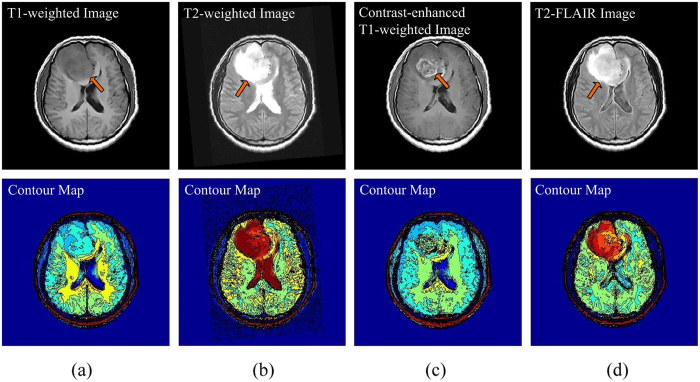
Representative brain tumor MR images under different imaging modes (upper) and the corresponding contour maps (lower). (a) T1-weighted image. (b) T2-weighted image. (c) Contrast-enhanced T1-weighted image. (d) T2-FLAIR image.
